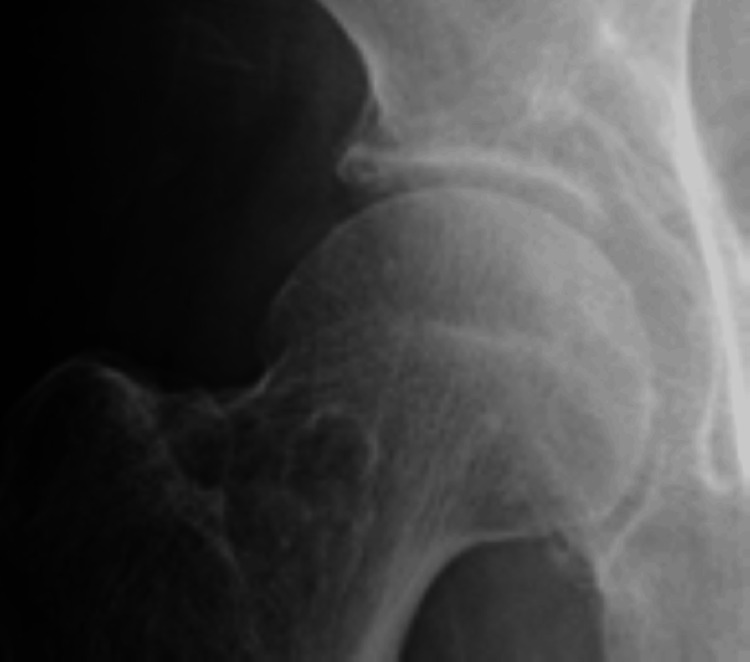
Anteroposterior radiograph of the right hip after 1 month of administration demonstrated disappearance of the calcification.
